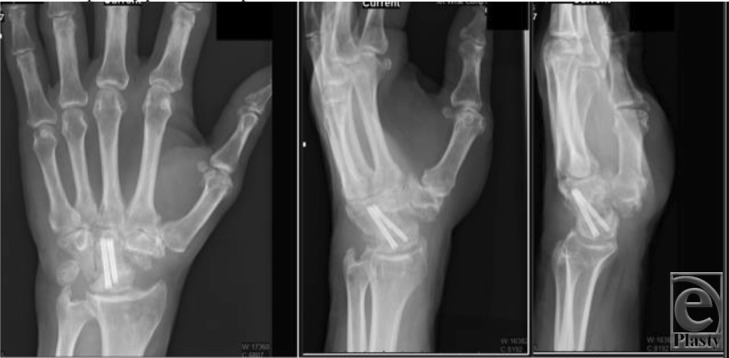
Postoperative posteroanterior, lateral, and oblique radiographs at 4 months revealing consolidation of primary partial midcarpal wrist arthrodesis.
